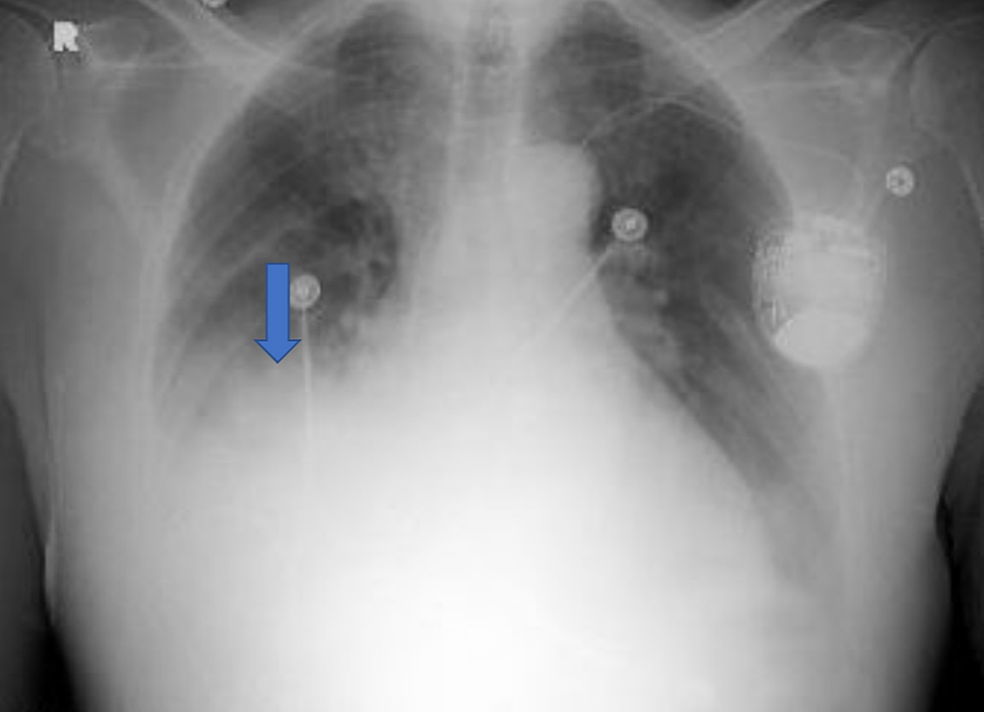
Chest X-Ray Revealing a Right Middle Lobe Infiltrate (Blue Arrow).
